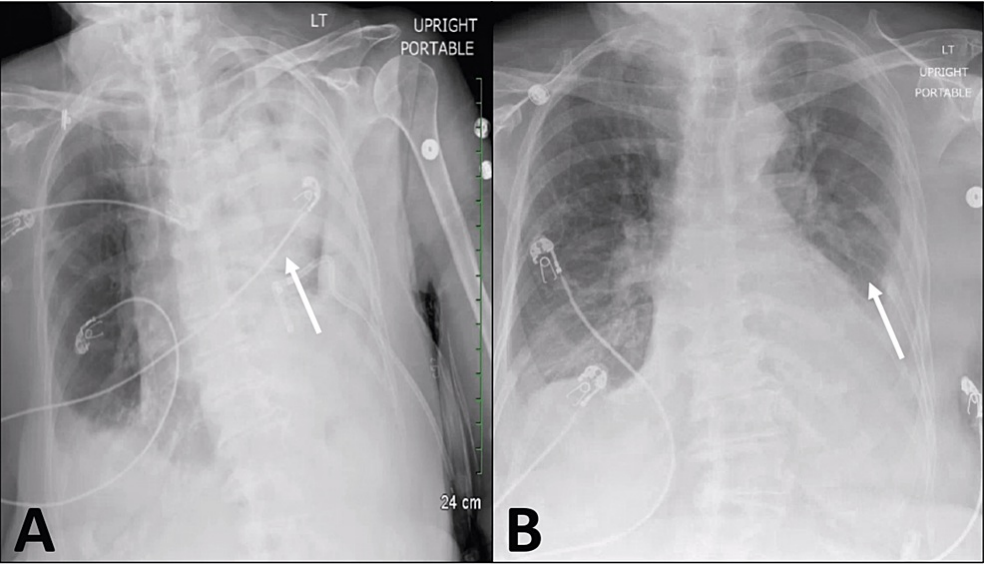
A). Anterior-posterior chest x-ray prior to scheduled video-assisted thoracostomy showing bilateral congestion and large left effusion, indicated by the white arrow. B). Anterior-posterior chest x-ray taken after video-assisted thoracostomy showing near resolution of the left-sided effusion and full left lung expansion, white arrow indicating the normal lung parenchyma.
